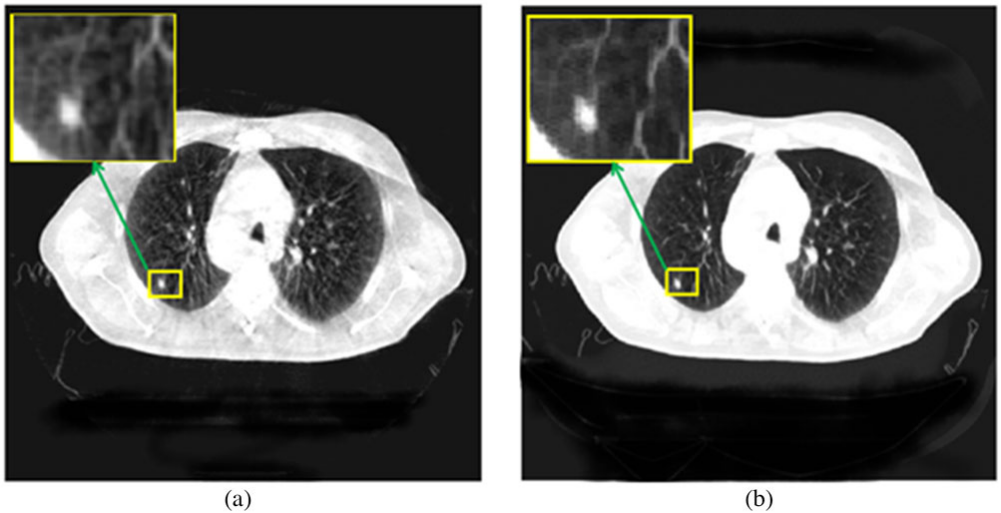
Spiculation tumor. Display window [−100,1063]. (a) The original CBCT image; (b) the correction result of our proposed method.
